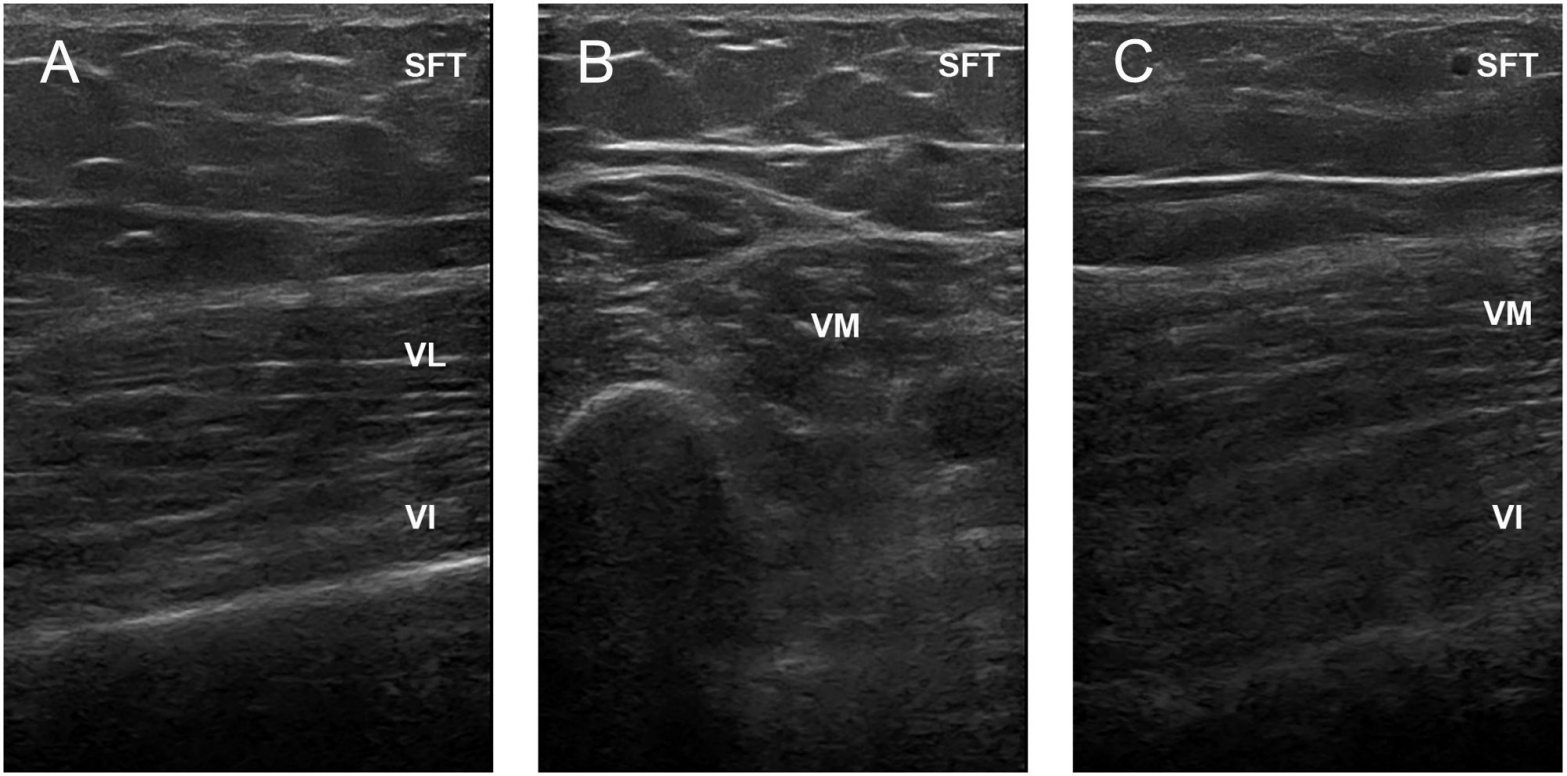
Examples of muscle ultrasound images in the CTM group. A. Longitudinal section of the VL. B. Cross section of the VM. C. Longitudinal section of the VM. CTM, chronic thyrotoxic myopathy; SFT, subcutaneous fat thickness; VI, vastus intermedius; VL, vastus lateralis; VM, vastus medialis.
